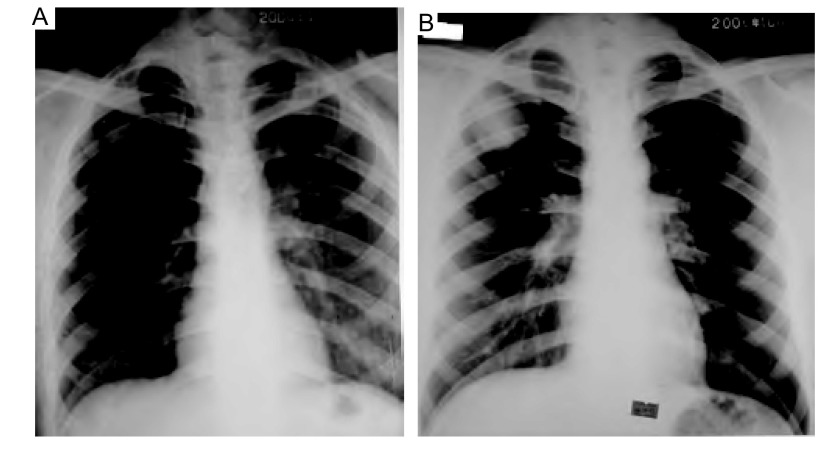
不同时期的胸片。A图显示常规体检时发现的右上叶片状影，B图示抗结核治疗后右上叶的团块影较前增大。 Chest X-ray of different period. A showing a patch lie in right upper lung when routine examing, and B showing a mass in the same place is larger than befor after anti-tuberculosis theraping.
